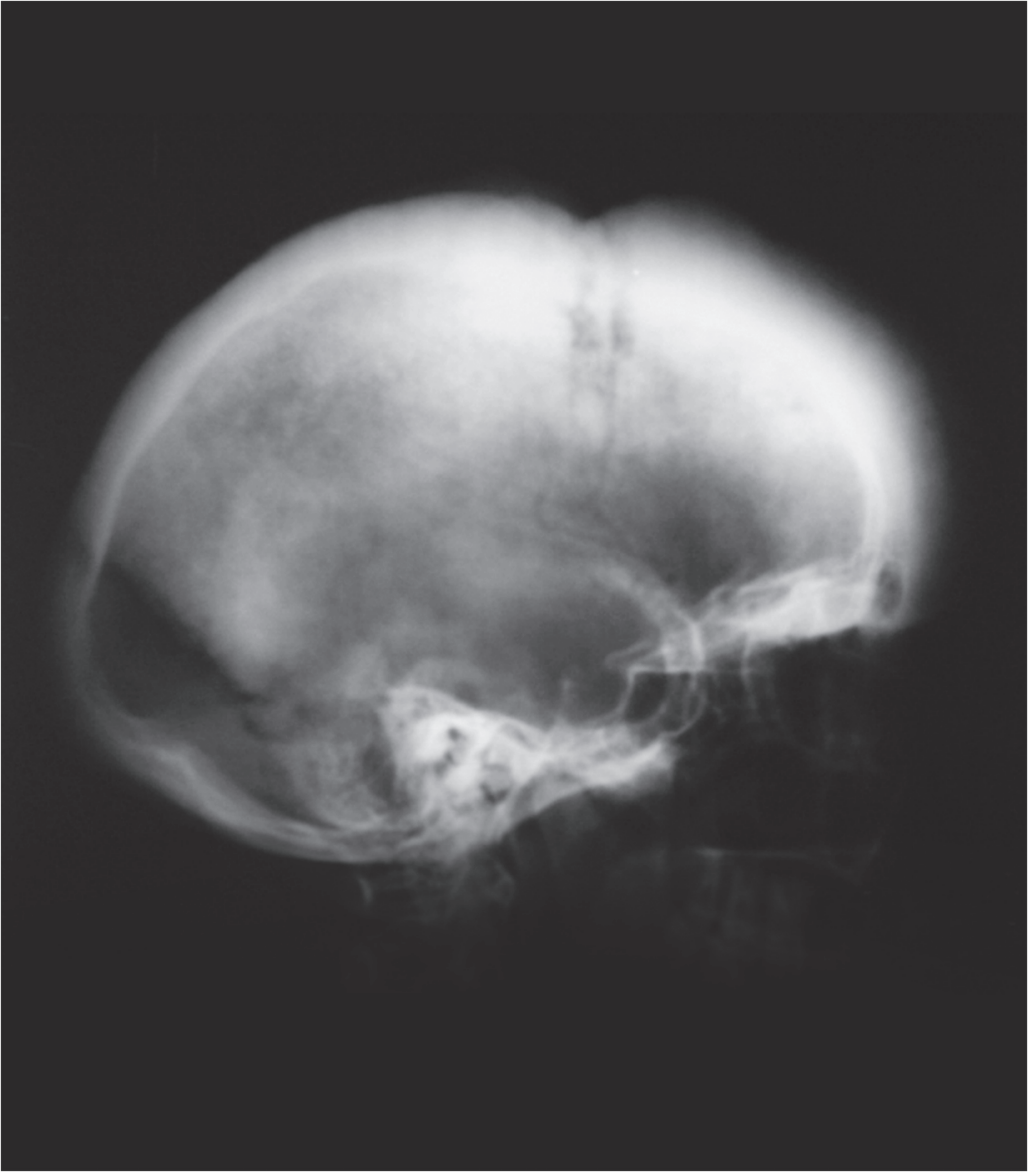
Skull X-ray showing massive thickening of the skull tables and generalised osteoblastic changes.
